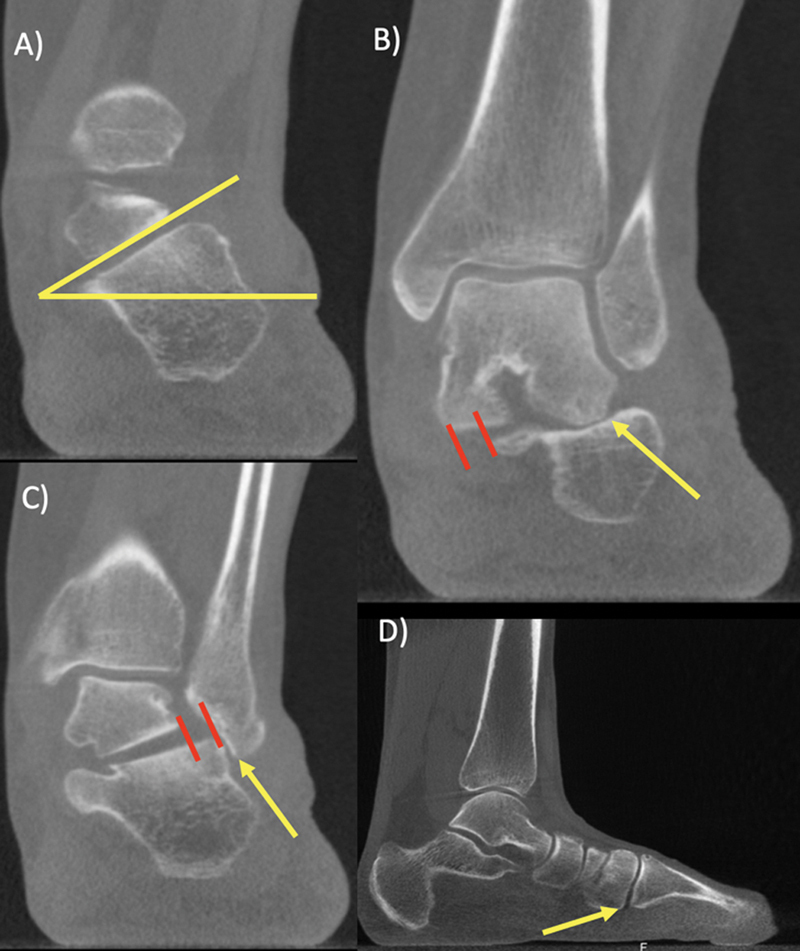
Coronal weight-bearing computed tomography scan demonstrating: (
